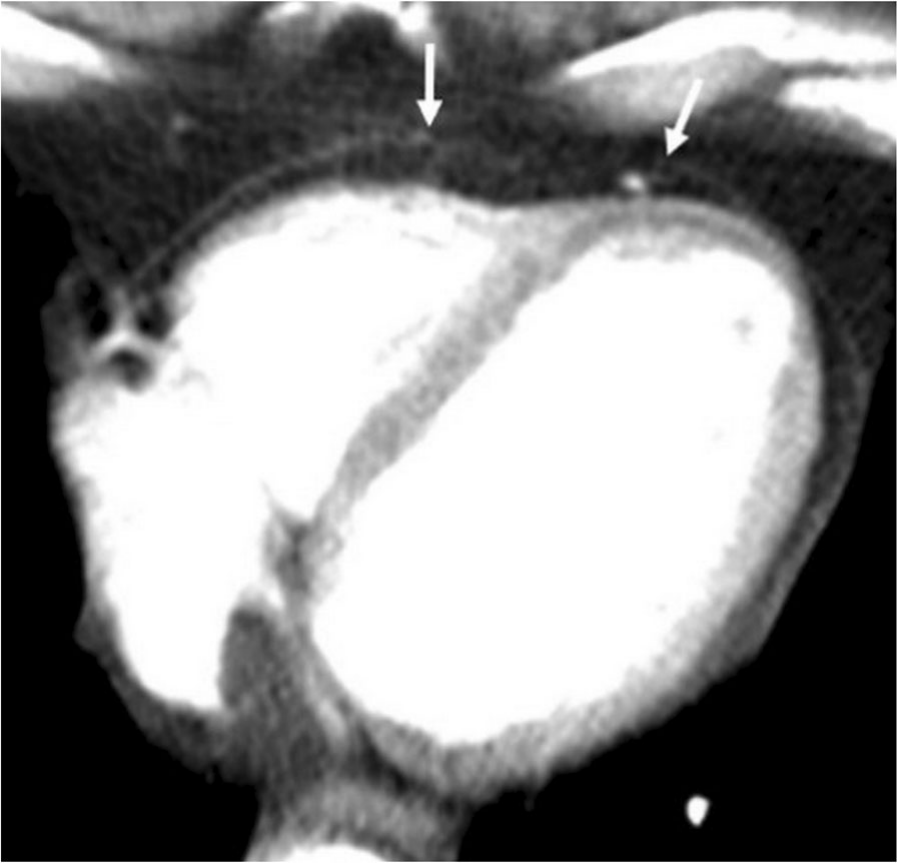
A 68-year-old man with previous coronary artery bypass surgery. Axial contrast-enhanced CT image demonstrates the lack of pericardial continuity (arrows) due to surgery
